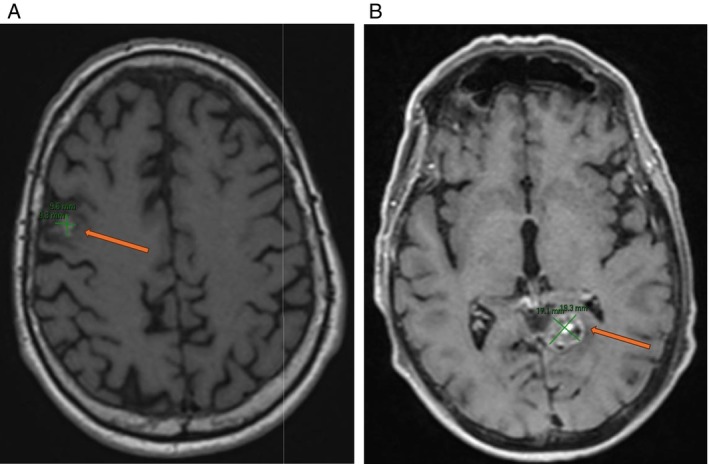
(A) MRI‐B. 10 × 9 mm lesion in the right middle frontal gyrus centred at the grey‐white matter junction. The lesion demonstrates intrinsic T1 hyperintensity with no appreciable enhancement. There is surrounding T2/FLAIR signal hyperintensity involving the subcortical white matter and cortex. There is extensive SWI signal abnormality corresponding to calcification on prior CT. (B) MRI‐B. 19 mm medial left occipital lobe metastasis with mild vasogenic oedema.
